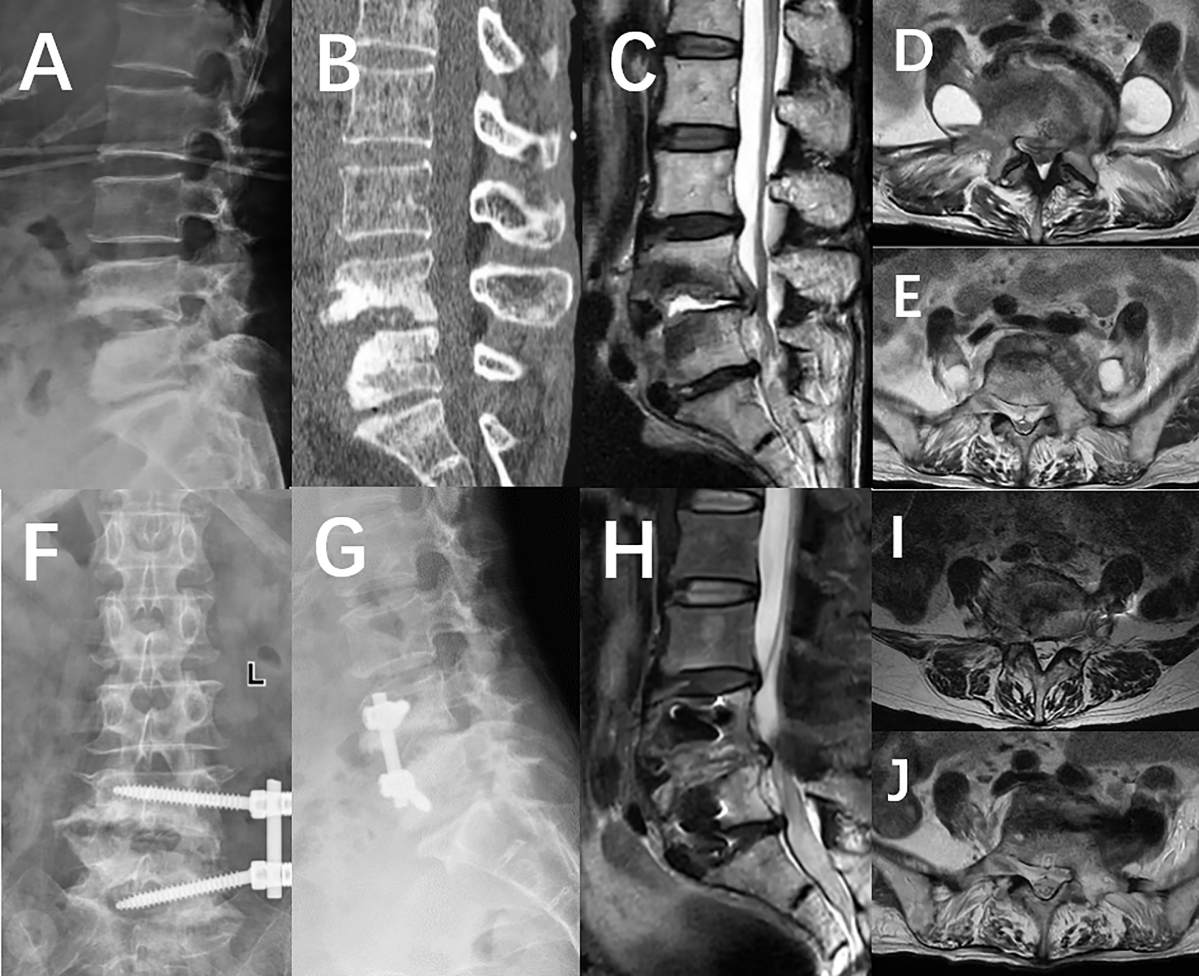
(A) X-ray imaging demonstrated destruction of the L4 and L5 vertebral bodies, as well as a decrease in the intervertebral space height. (B) The CT scan revealed a significant amount of bone erosion in the L4 and L5 vertebrae, resembling the effects of a worm-eaten surface. (C) Magnetic Resonance Imaging demonstrated the formation of an abscess in the L4/5 intervertebral space, in the spinal canal, and around the vertebral body. (D, E) Abscesses accumulated in the psoas bilaterally and spread caudally along the intermuscular space. (F, G) An X-ray taken a week after the operation revealed that the internal fixation was in the correct position. (H–J) The postoperative MRI indicated that the abscess had been completely drained and cleared after the Negative Pressure Wound Therapy treatment.
